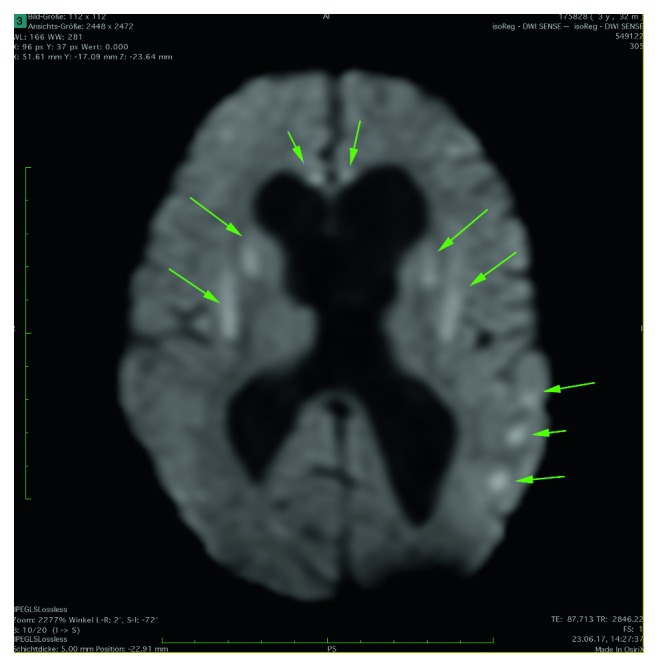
DWI—bilateral diffusion impairment in the basal ganglia, corpus callosum, and subcortical regions with slightly dilated lateral ventricles.
